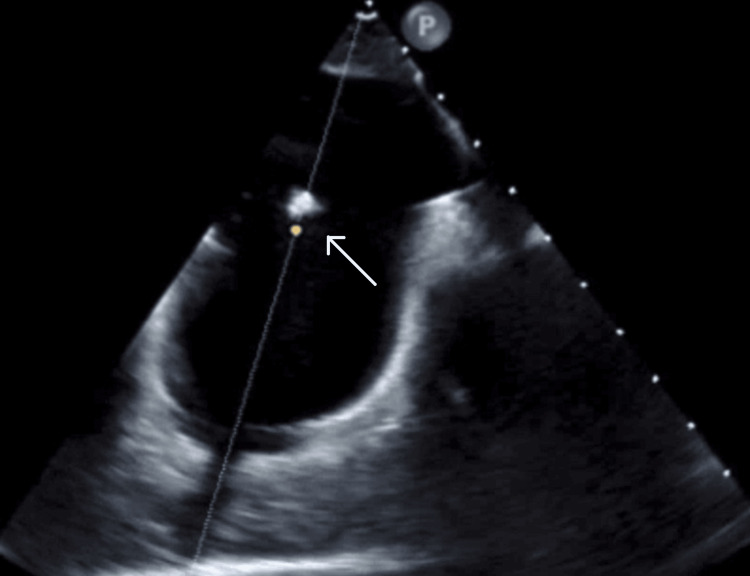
X-plane transthoracic echocardiography (TEE) showing echodensity attached to Chiari network Transthoracic echocardiography showing a mobile echodensity within the right atrium (white arrow), attached to a filamentous structure consistent with a Chiari network, raising concern for superimposed vegetation.
